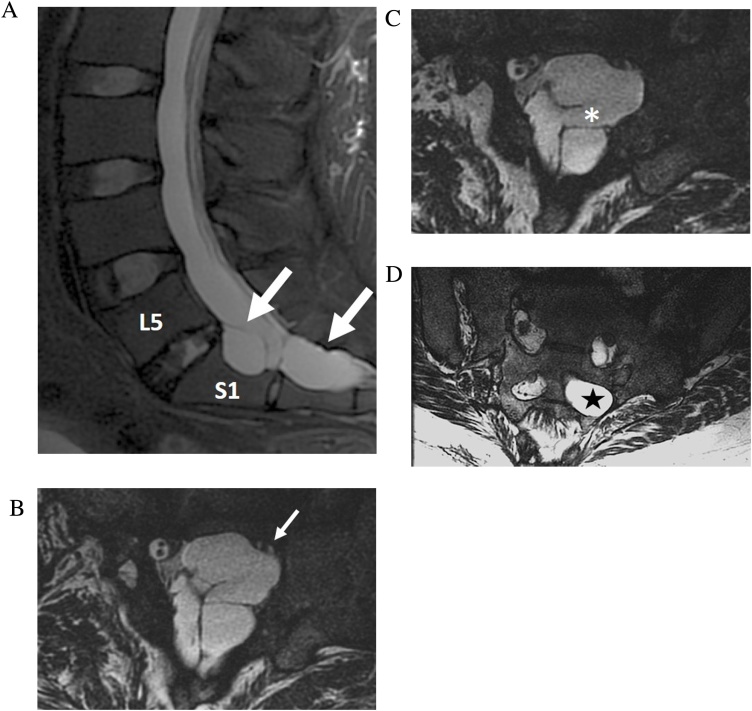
Post traumatic pseudomeningocele with nerve root avulsion. 25-year-old woman with chronic bowel and bladder incontinence and left leg pain after motor vehicle accident. Sagittal T2-weighted FSE MRI (a) shows a complex multiseptated post traumatic pseudomeningocele within the left S1 and S2 neuroforamen. Sequential axial FIESTA-C MRI images (b–d) resolve the site of dural tear (asterisk), the compressed left S1 nerve root within the S1 neuroforamen (small arrow), and absence of the left S2 nerve root (star) due to avulsion.
